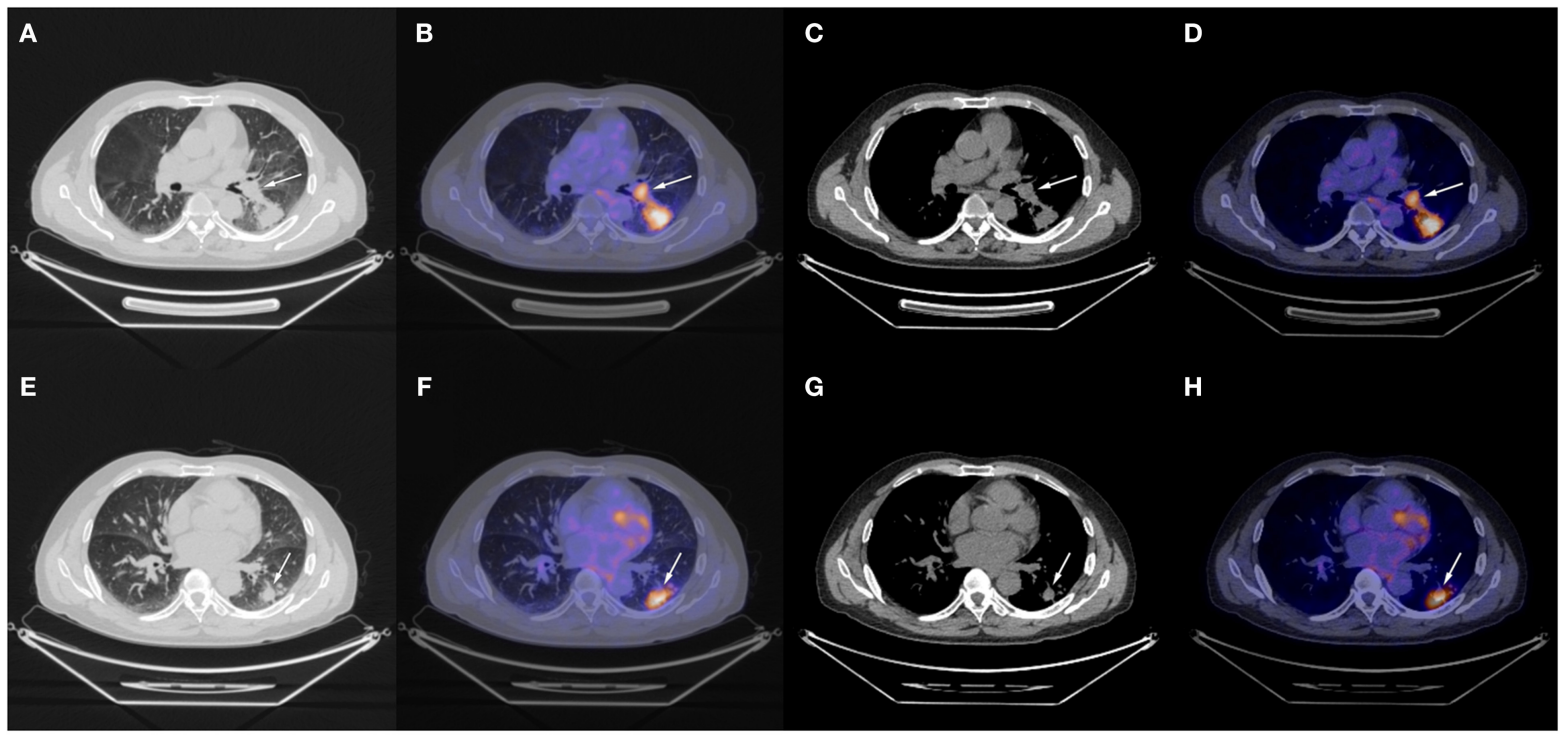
Chest CT scan and fused PET-CT images of the masses on April 28th, 2020. (A–D) The SUVmax of the mass adjacent to the hilum was 8.4 and the size of it was 1.7 cm × 2.3 cm × 2.0 cm; (E–H) The SUVmax of the mass on the basal segment was 8.4 and the size of it was 1.7 cm × 1.8 cm × 1.6 cm. White arrows indicate the locations of the masses.
